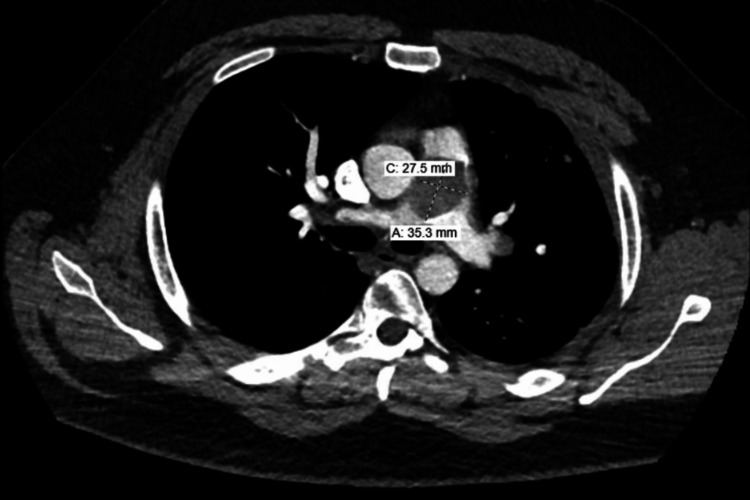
Pulmonary mass on CT imaging
